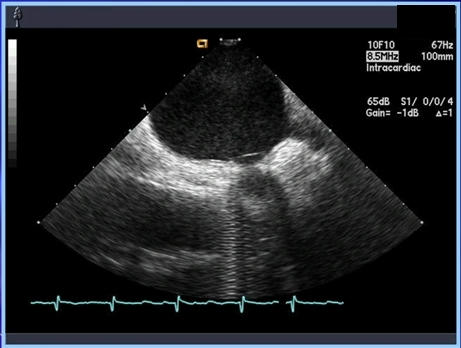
Intracardiac ultrasound image obtained of the left atrium showing a circumferential mapping catheter at the ostium of the left lower pulmonary vein. Accurate knowledge of the pulmonary vein ostium in children is critical and can be difficult to ascertain with standard fluoroscopy.
